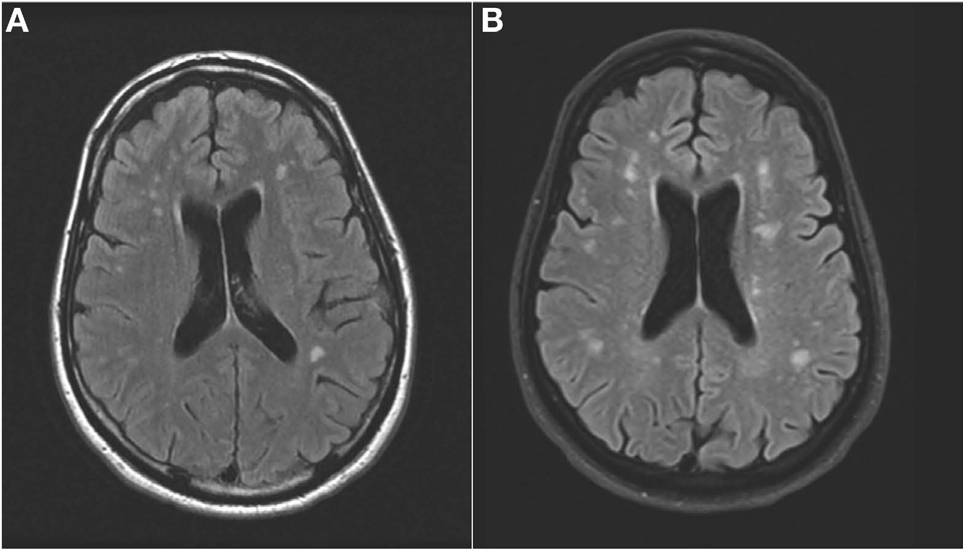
(Axial FLAIR): hyperintense signal in the periventricular white matter that increased from prior to the event (A) to the time of the acute event (B).
